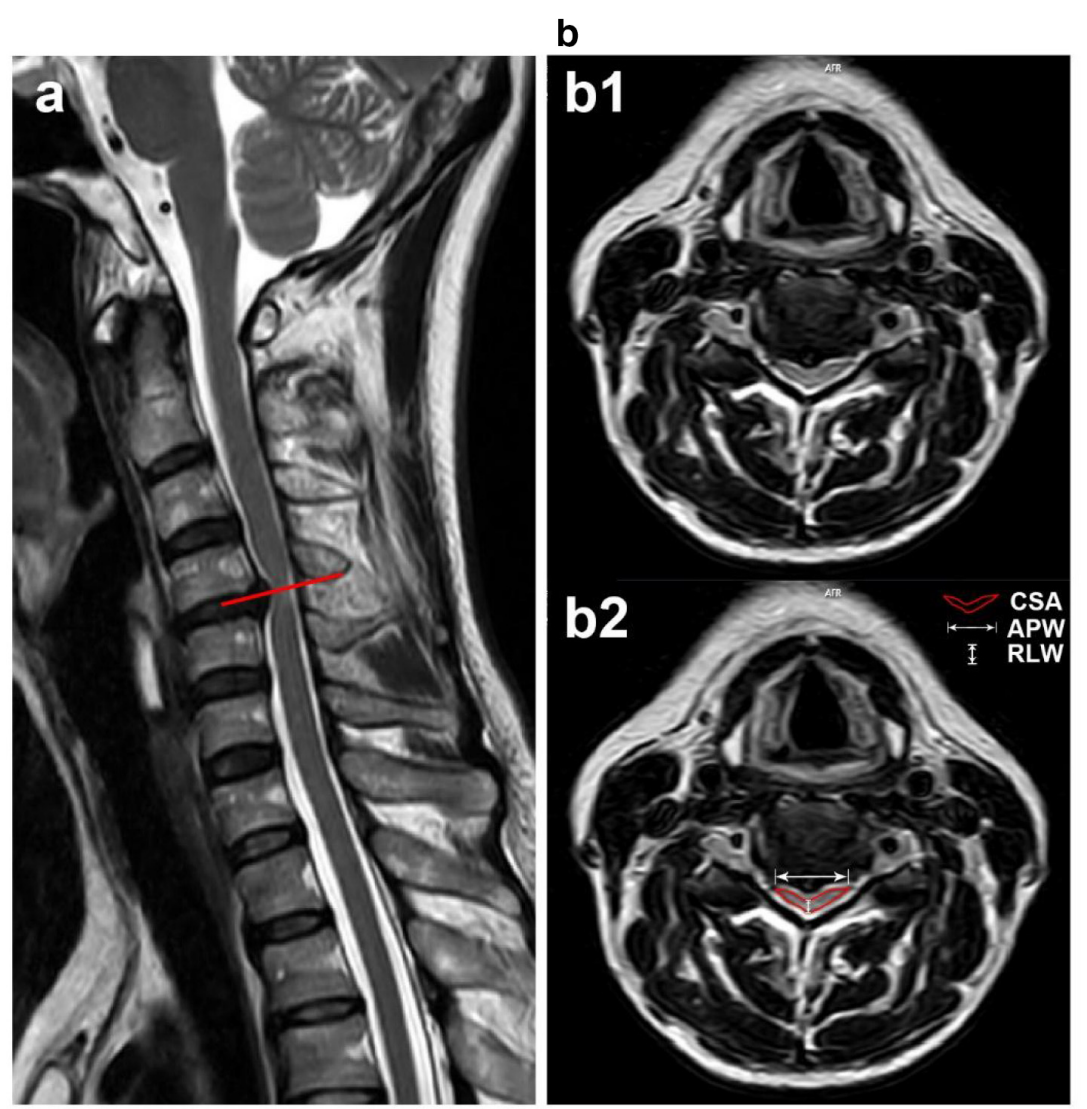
A T2-weighted MRI depicting a 53-year-old female with confirmed DCM. She complained of hand clumsiness and limb numbness for 6 months, with a total mJOA score of 14. Characteristics of the compressed cervical cord are shown: (a) Sagittal view showing C4/5 stenosis (indicated by the red line). (b) Transverse view (b1) showing the slice with the most severe cervical cord compression, with CSA, APW, and RLW marked in (b2).
